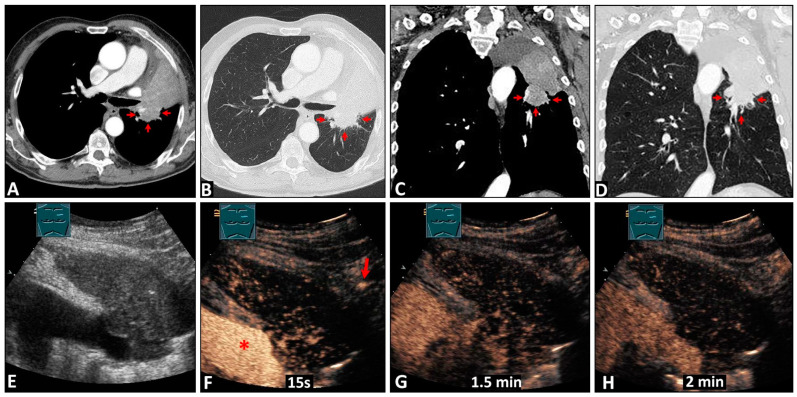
A 75-year-old patient with a bronchoscopically confirmed squamous cell carcinoma of the left upper lobe. On computed tomography scans (A–D), a central tumor formation (arrows) with obstructive atelectasis is visible. On B-mode lung ultrasound (E), a central tumor is not distinguishable from the atelectatic tissue. On contrast-enhanced ultrasound, the atelectatic tissue shows homogeneous delayed bronchial arterial enhancement simultaneously with the chest wall (arrow) after the enhancement of the pulmonary artery (*), which is a sign of systemic vascularization (F). After 1.5 min, a tumor formation is still not distinguishable (G). The atelectasis shows rapid washout after 2 min (H).
